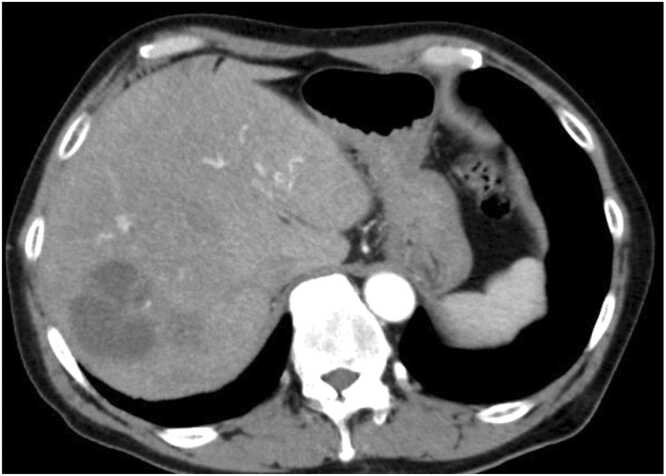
A contrast-enhanced CT scan of the abdomen. A multiloculated, 5 cm hypoattenuating lesion in the right hepatic lobe is noted, consistent with a liver abscess.
